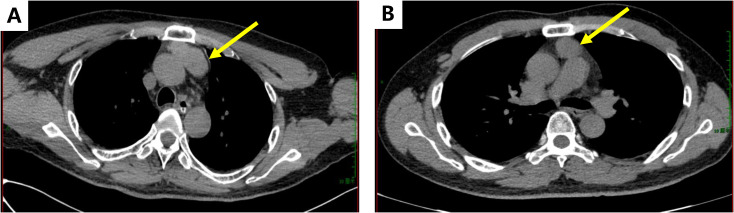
Chest CT. (A) Image on August 9, 2023. A patchy soft tissue density shadow appeared in the anterior superior mediastinum, which may be considered as thymoma or thymic hyperplasia. (B) Image on November 22, 2023. The mass soft tissue density shadow of the anterior superior mediastinum was thrown to consider the possibility of thymoma, but it was significantly smaller than before.
